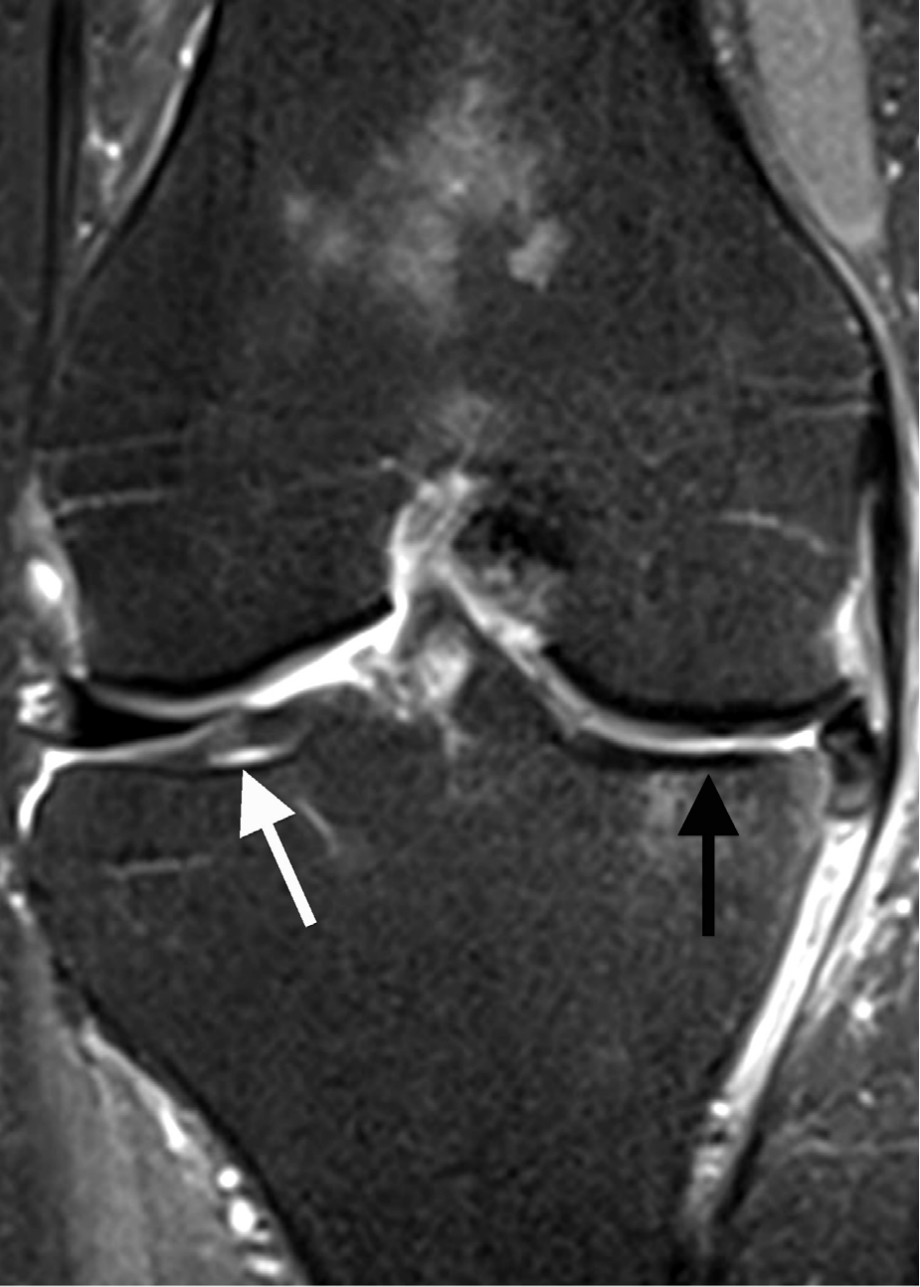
Coronal PD-weighted TSE MRI of a 68-year-old woman. Tibial medial MRI shows a full-thickness defect of the cartilage with denudation of the bone (black arrow). This finding is defined as a grade 4 disorder. Signal heterogeneities within the cartilage at the lateral tibia were documented as a grade 1 cartilage disease (white arrow).
